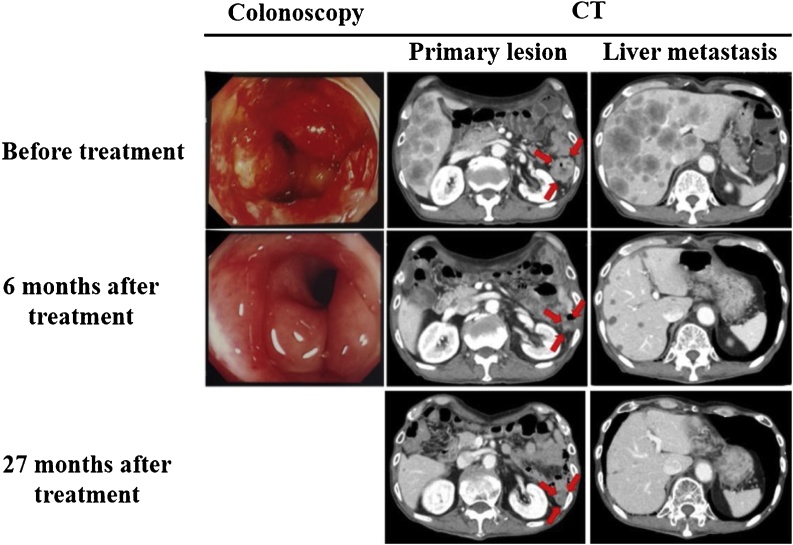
The occlusion of the descending colon improved, and no malignant findings were observed in the biopsy. CT scan also showed marked decrease in lesions, particularly, liver metastasis.
